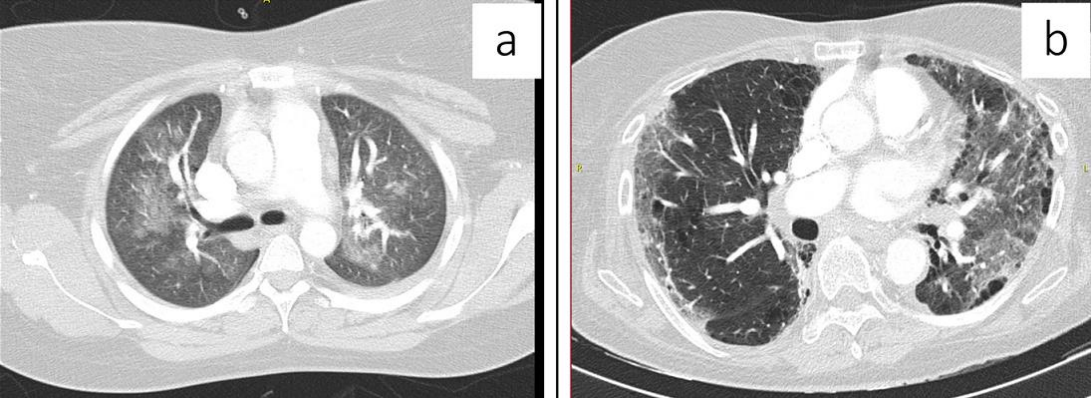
a) CT axial lung window demonstrating central distribution of ground glass changes; b) axial lung window CT demonstrating predominantly peripheral mixed reticulation and ground glass opacification
